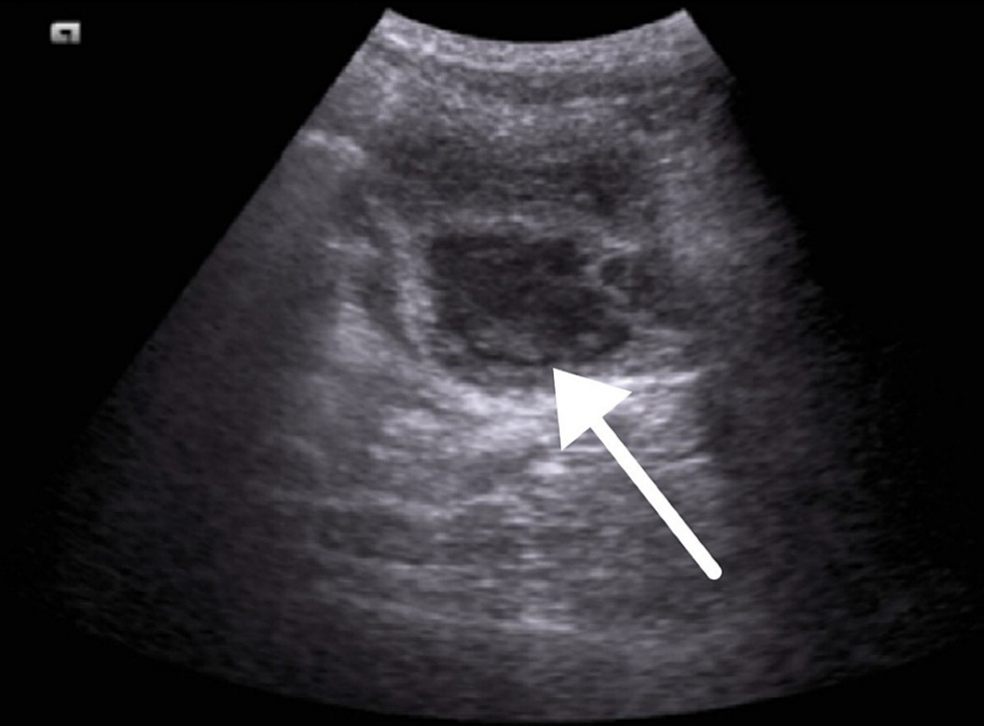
Ultrasound of the bladder revealing a well-defined, thick-walled hypoechoic lesion posterior to the urinary bladder measuring 5.3 × 3.7 cm
